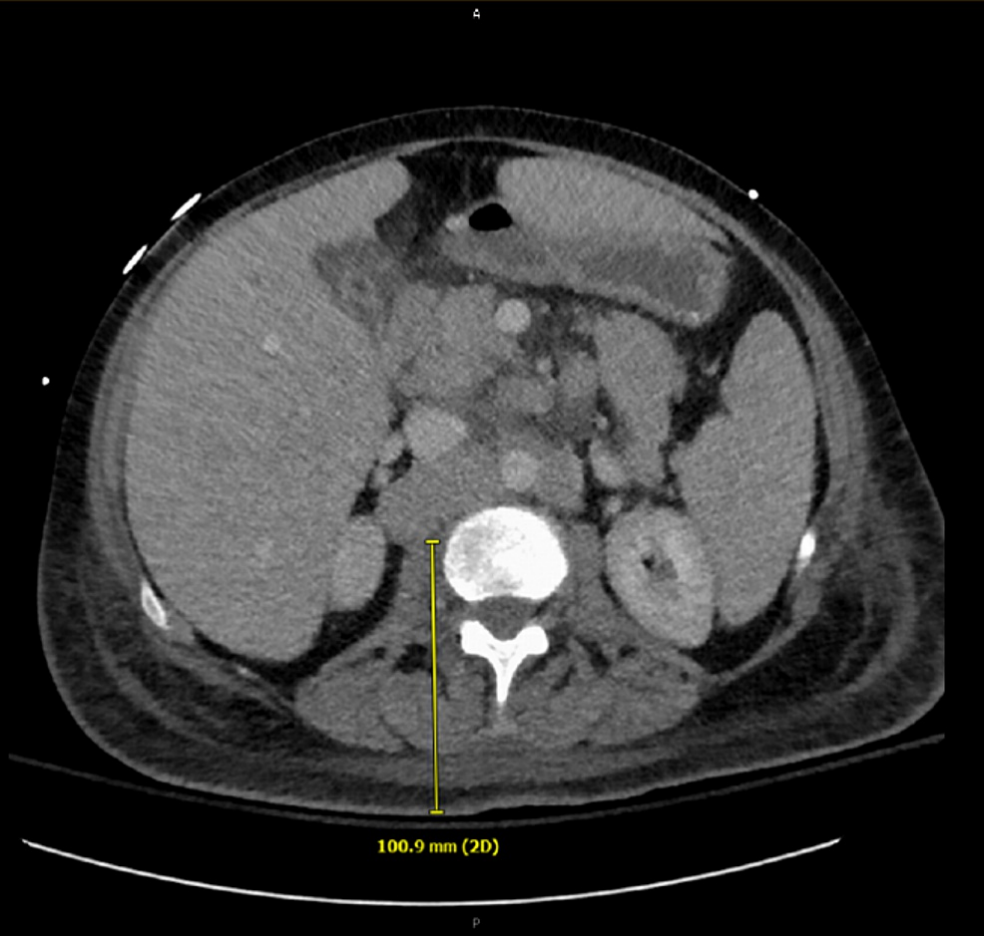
CT abdomen pelvis with and without contrast showing hepatomegaly, splenomegaly, and diffuse retroperitoneal lymphadenopathy
